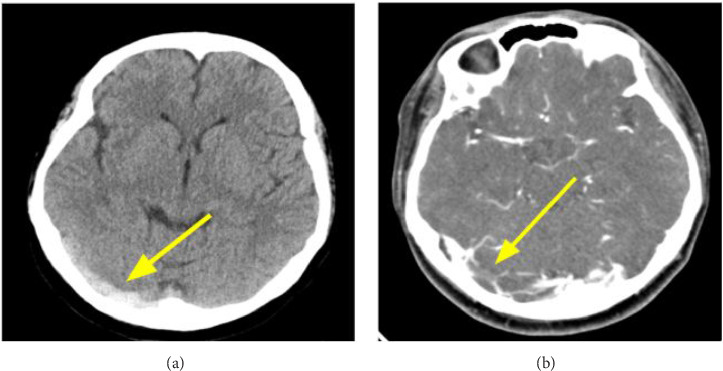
(a) Noncontrast-enhanced computed tomography of the head showing dense clot sign of right transverse sinus (yellow arrow). (b) Computed tomography venography showing small focal filling defects in the right transverse sinus, suspicious of thrombus (yellow arrow).
